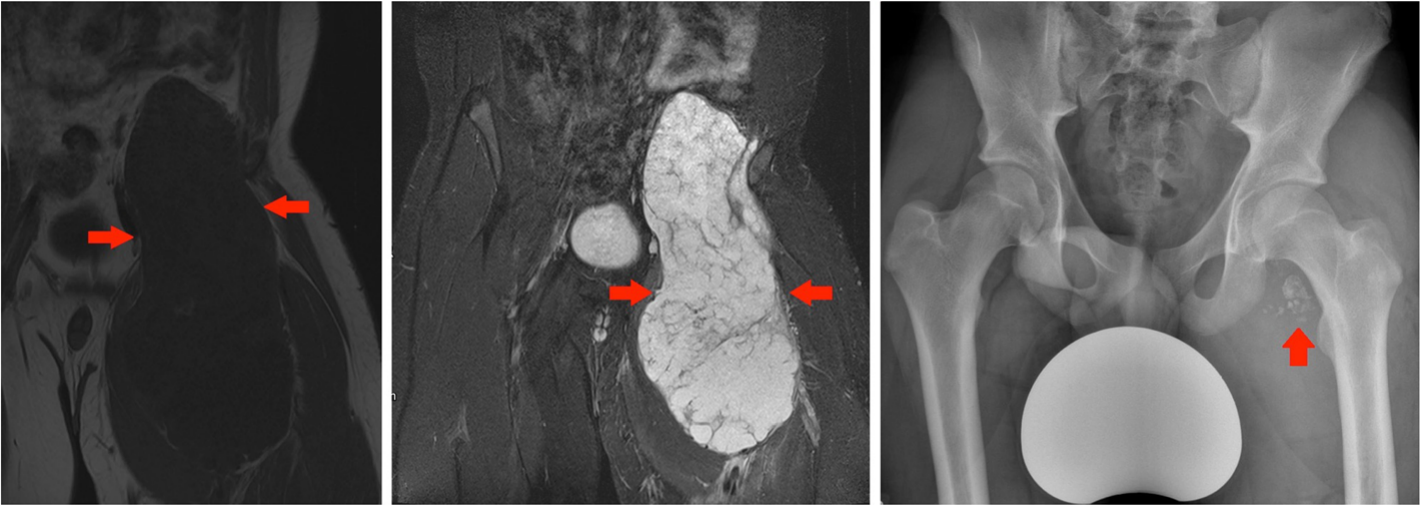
Left panel: Coronal T1-weighted image of the pelvis and upper thigh: large low signal intensity mass is seen in the left iliopsoas compartment (red arrows). This demonstrates a paucity of fat signal. Middle panel: Coronal T1 TIRM image of the pelvis and upper thigh: a high signal intensity is seen in the tumour with low signal septations and an overall aggressive appearance. Right panel: AP X-ray of the pelvis: red arrow confirms an area of intra-lesional chondroid calcifications. Biopsy confirmed a high-grade chondrosarcoma. Intra-operatively the tumour appeared to be originating from the lesser trochanter
